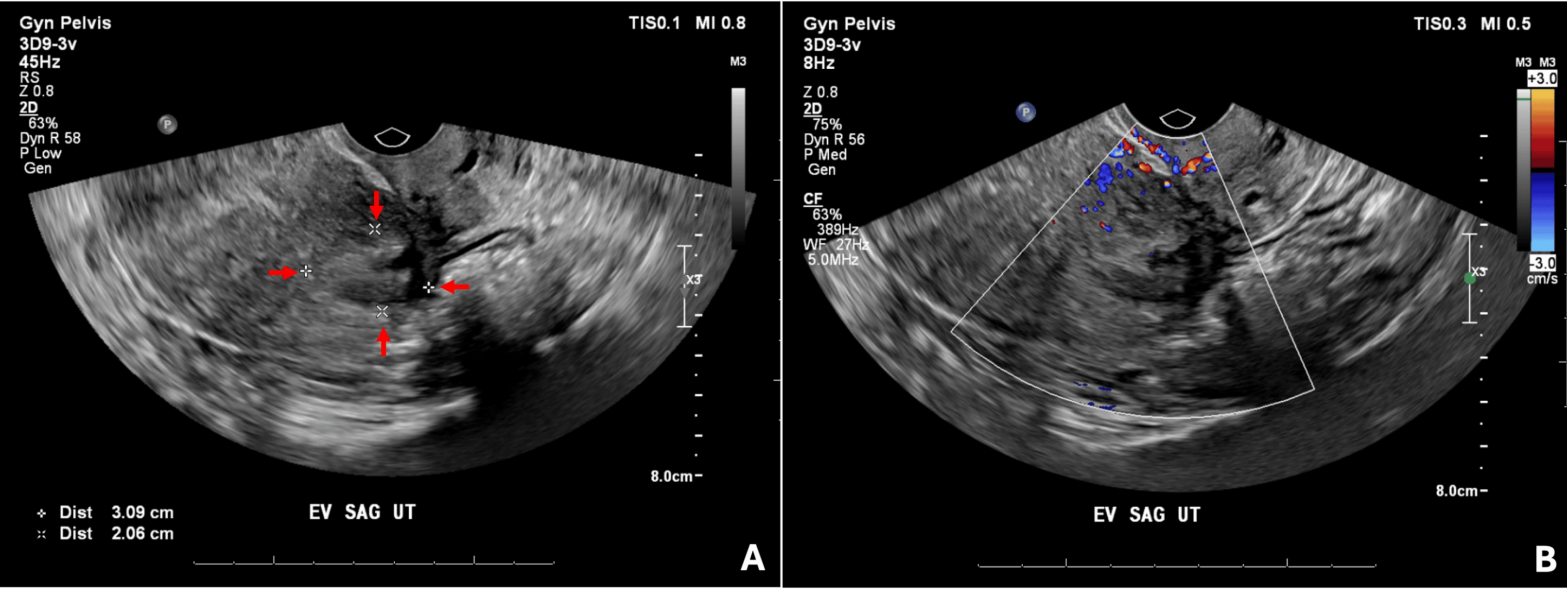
Transvaginal ultrasound imaging of the uterine leiomyoma. Images demonstrate the 3.09 cm x 2.06 cm x 2.08 cm intramural uterine leiomyoma in the lower uterine segment. (a) Fibroid outline from a sagittal image of the uterus indicated by the red arrows. (b) Fibroid on a sagittal image of the uterus with color Doppler.
